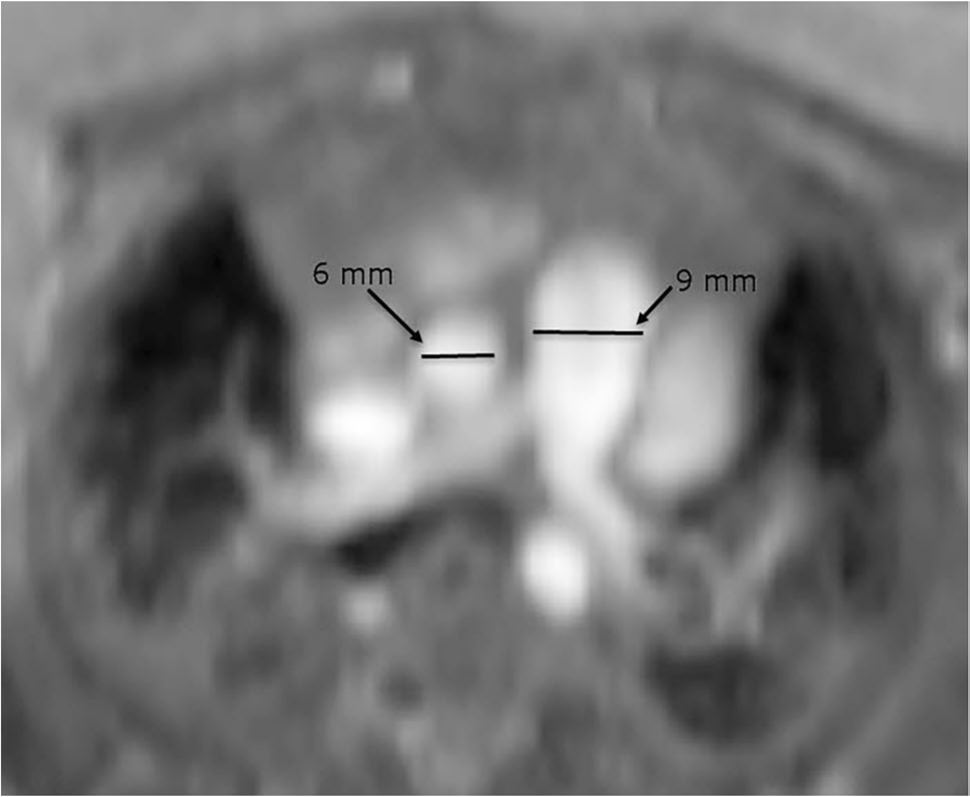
Morphological markers of pulmonary hypertension in a girl born at 26 weeks of gestation with MRI performed at post-menstrual age 38 weeks (12 weeks old). Axial MRI bright-blood image through the main pulmonary artery and the ascending aorta demonstrates a 9-mm main pulmonary artery and a 6-mm aorta, resulting in a ratio of 1.5:1. A ratio of 1.3:1 or greater, in the absence of a larger left-to-right shunt, is very specific for pulmonary hypertension
